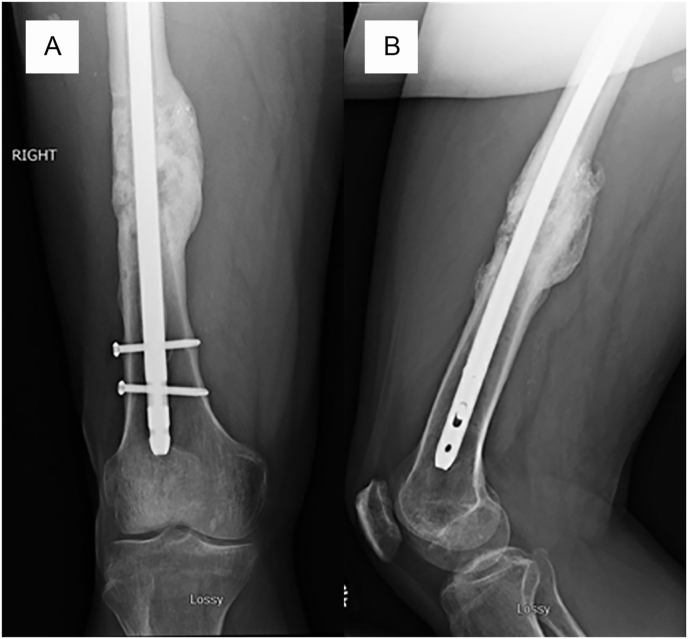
Anteroposterior (A) and lateral (B) right femur radiographs demonstrating bridging callus in 3 of 4 cortices with no evidence of catastrophic hardware failure of the antibiotic coated intramedullary nail.
